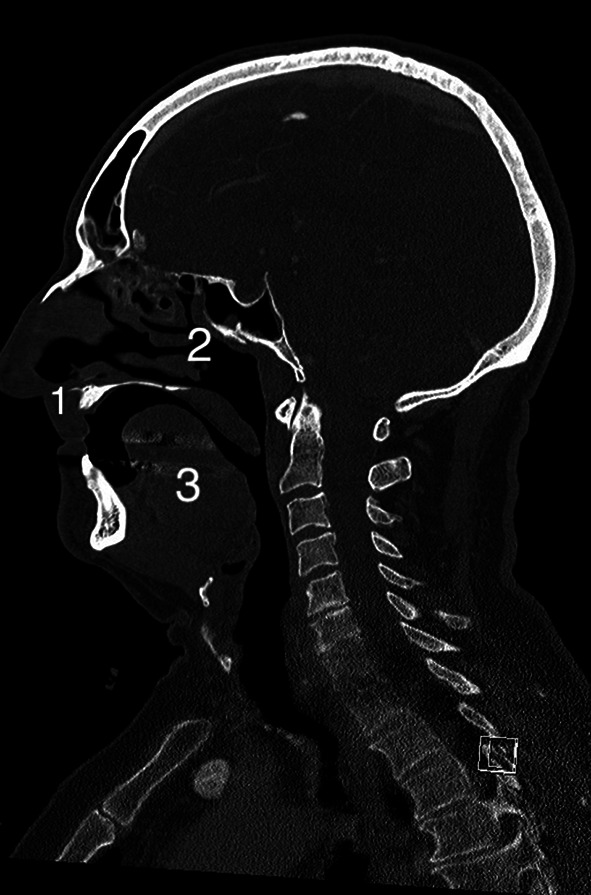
CT-scan of the head and representation of the right side of the face with recognisable anatomical structures of the nose (image taken on 11/03/2024). (1: hard palate; 2: nasal conchae; 3: tongue)
